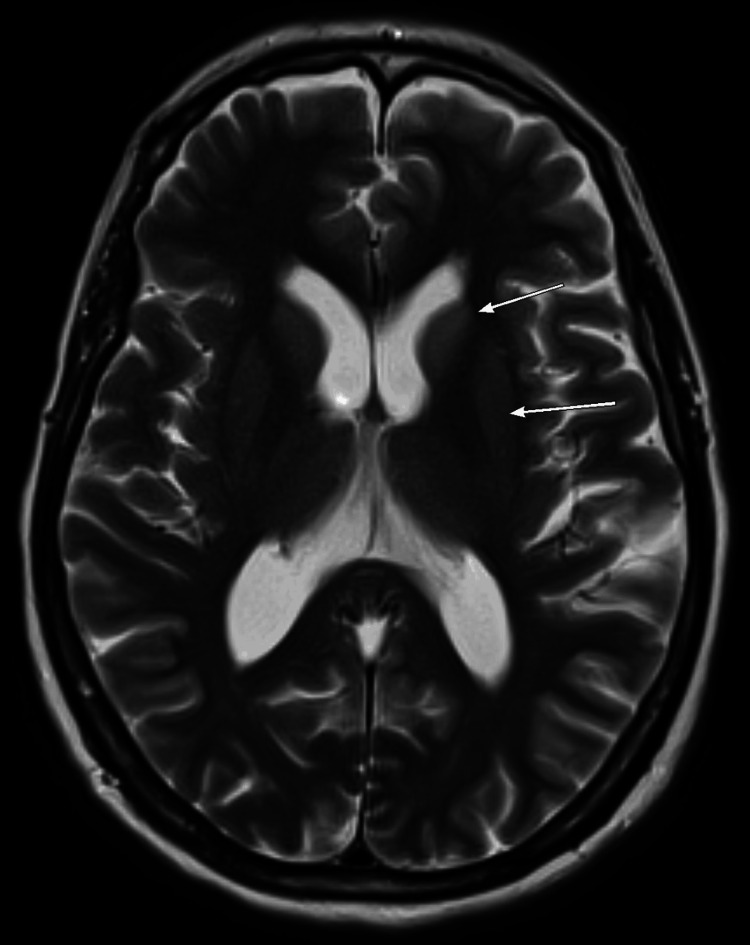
T2-weighted image showing signal alteration of the aforementioned deep gray matter (large arrows).
